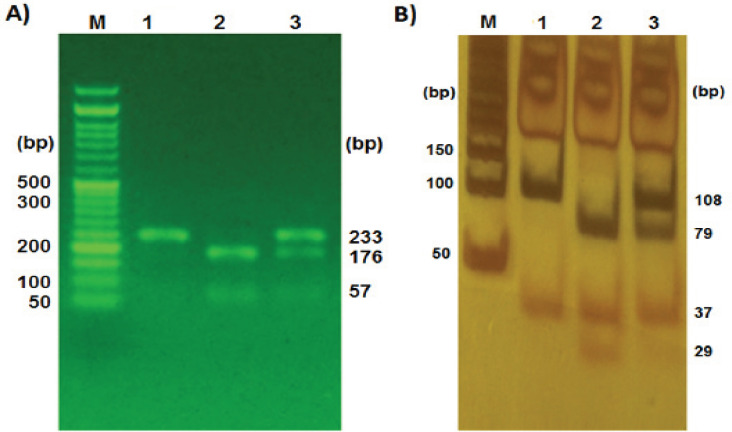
Polymorphism Analysis of *MTHFR*-C677T and *MTHFR*-A1298C. A) The PCR products containing C677T variation which digested with Hinf I restriction enzyme (lane M, DNA marker; Lane 1, CC genotype; Lane 2, TT genotype; Lane 3, CT genotype); B) The PCR products containing A1298C variation which digested with MboII restriction enzyme (lane M, DNA marker; Lane 1, CC genotype; Lane 2, AA genotype; Lane 3, AC genotype)
